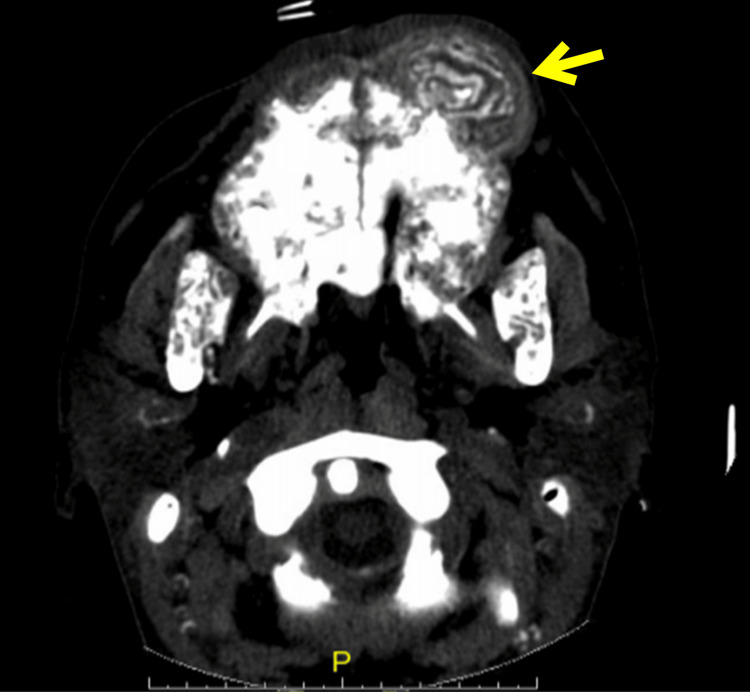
CT scan of the face and soft tissues showing a left maxillary mass (arrow) CT: computed tomography
